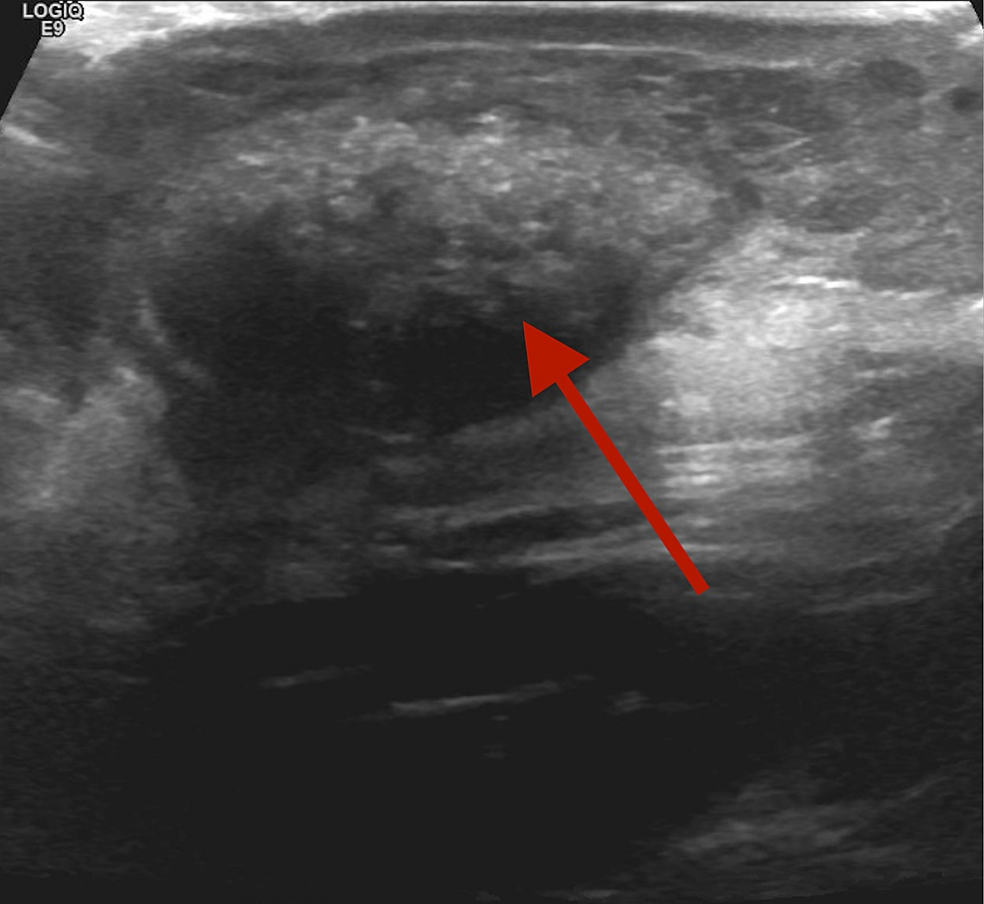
Ultrasound of the mass Ovoid heterogeneous mass involving the subcutaneous tissue of the posterior left shoulder with the associated crescentic hypoechoic area.
